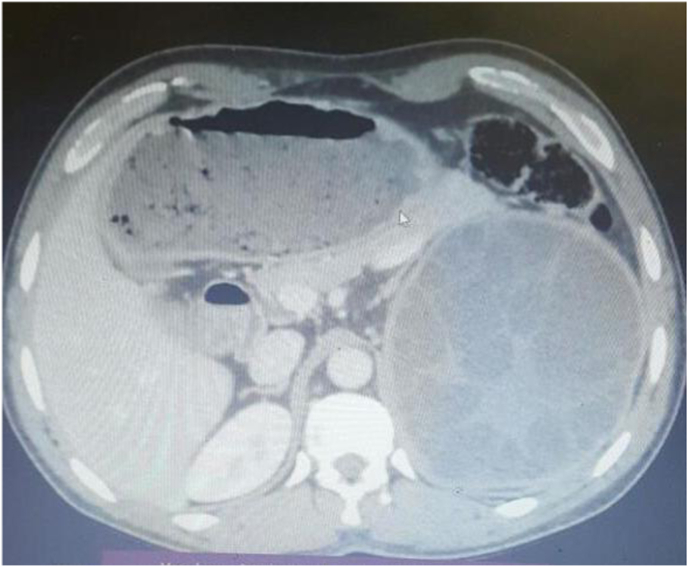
Pre-operative abdominal computed Tomography shows a mildly enhancing complex cystic mass with multiple peripheral small cysts and a central solid component situated in the superior part of the left kidney.
